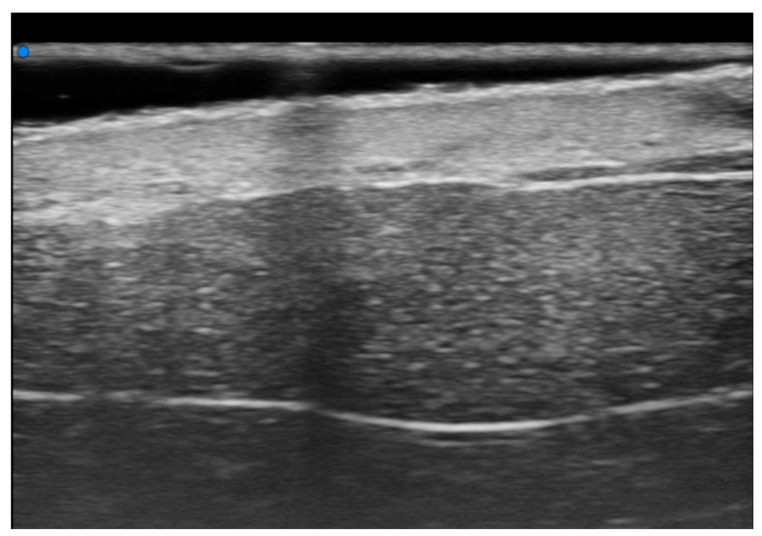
High-frequency ultrasound image of normal skin, showing a thin, continuous hyperechoic epidermal line and a homogeneous dermis with relatively lower echogenicity, without evidence of subepidermal low-echogenic band (SLEB) (UHF 70 MHz).
